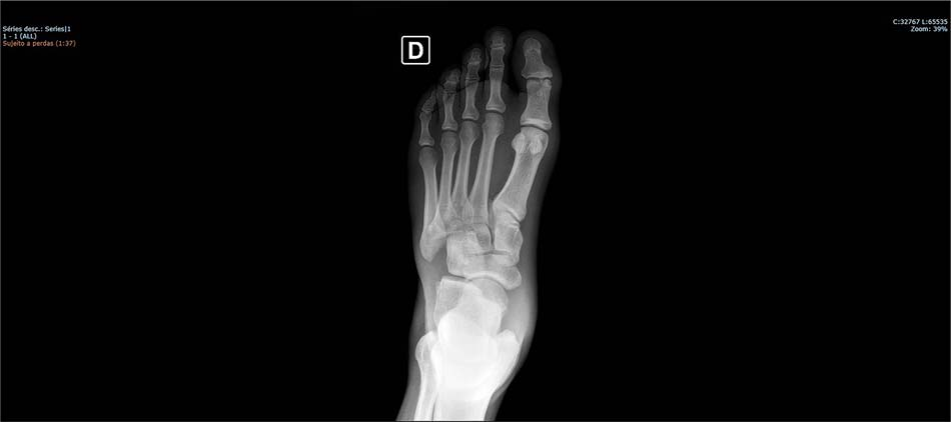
Preoperative X-ray of the foot in AP view
